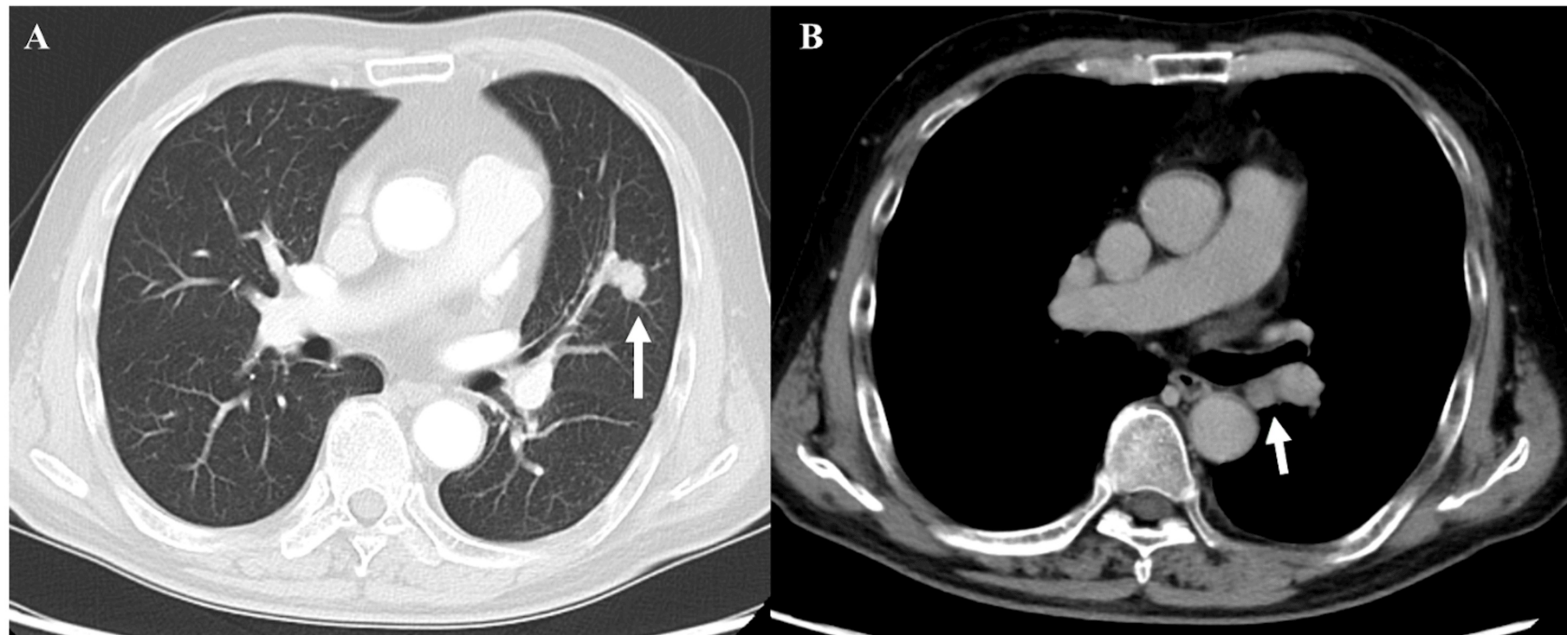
Preoperative CT findings. Axial view of the chest CT scan performed prior to surgery, revealing a nodule in the left upper lobe (A, indicated by the white arrow) and evidence of mediastinal lymph node metastasis (B, also indicated by the white arrow in the mediastinal window).
